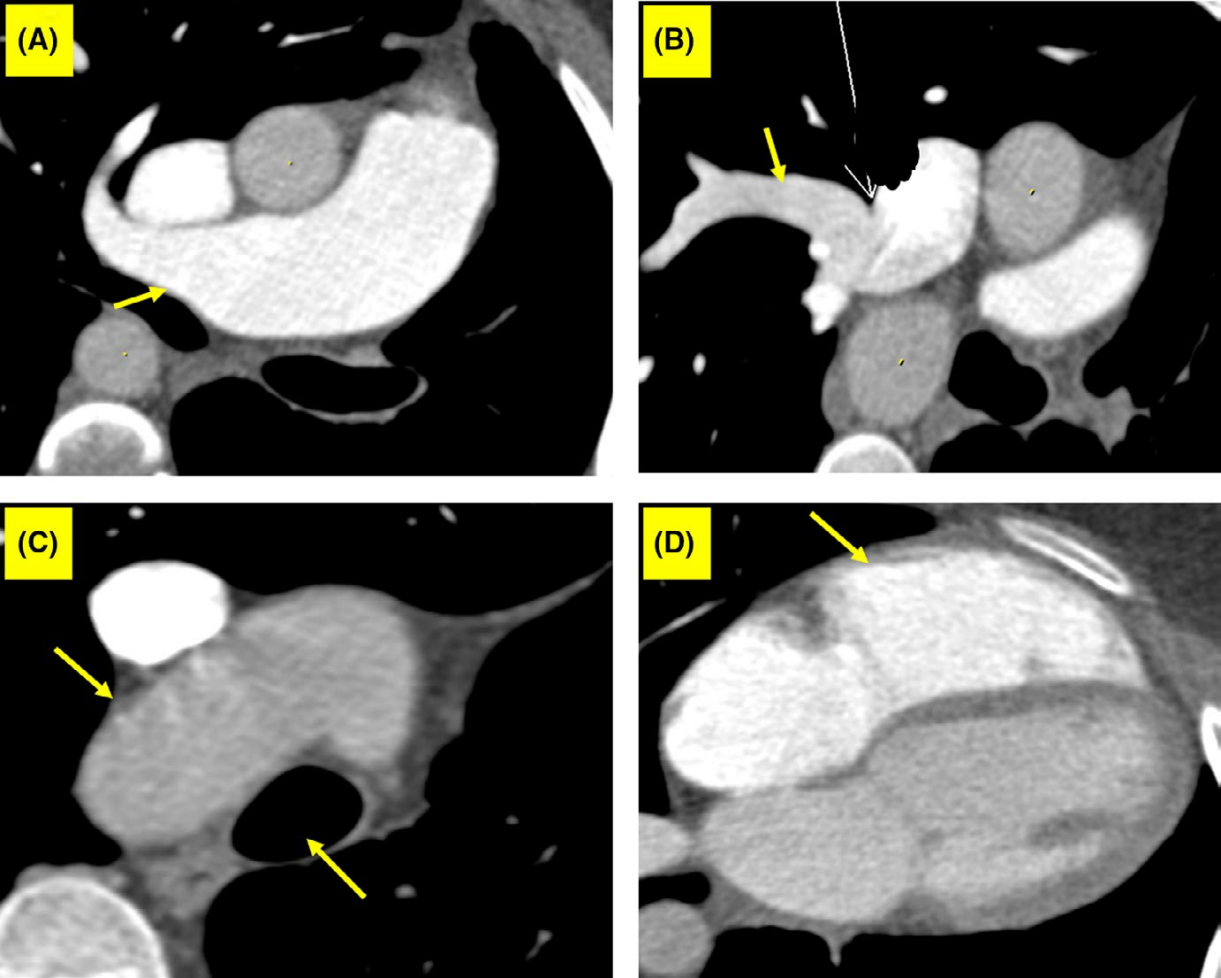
Computed tomography angiography views of the patient with UAPA show (A) absence of LPA with RPA dilation (arrow), (B) PAPVC between RUPV and SVC (arrow), (C) right‐sided aortic arch (upper arrow, with the lower arrow pointing to the trachea), and (D) mild RV enlargement (arrow) in the 4‐chamber view. Abbreviations: UAPA, unilateral absence of pulmonary artery; LPA, left pulmonary artery; RPA, right pulmonary artery; PAPVC, partial anomalous pulmonary venous return; SVC, superior vena cava; RV, right ventricle
